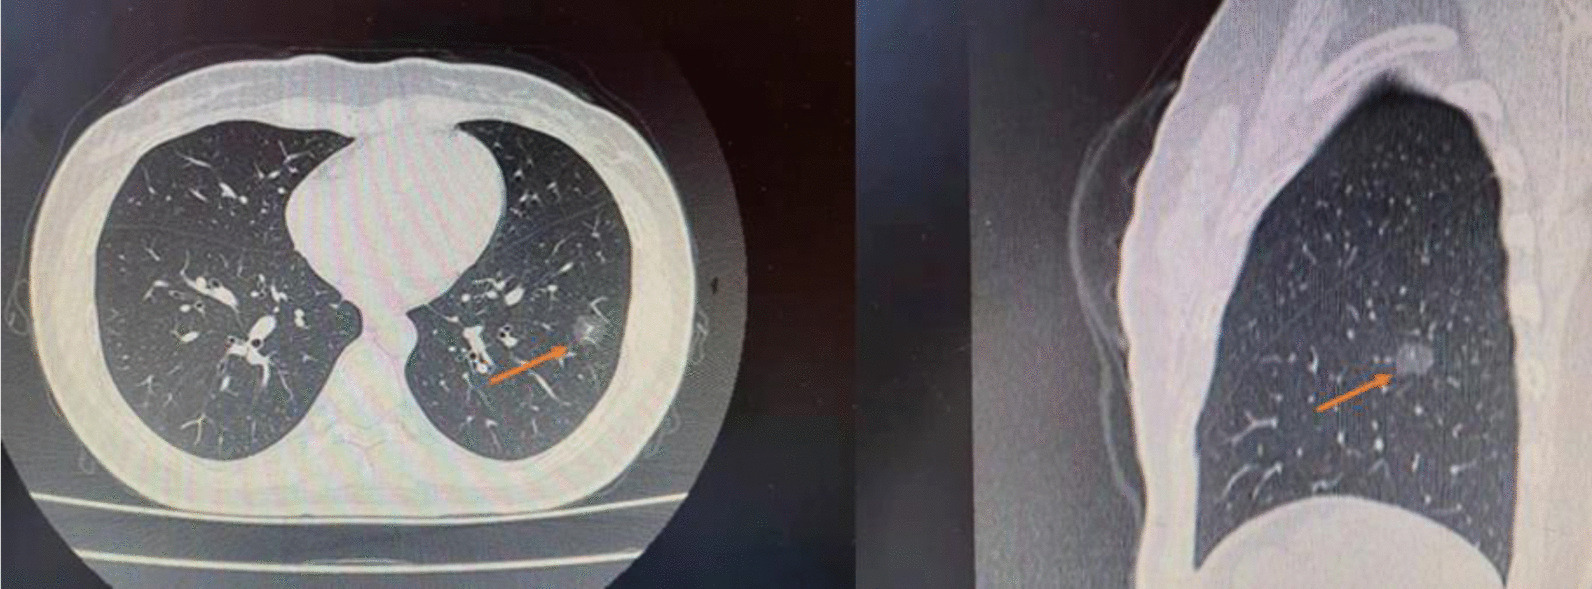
Computed tomography showed a 12 mm mixed ground glass opacity nodule (arrow) that was centrally located in the number 8 segment of the left lung (Left, axial plane; Right, sagittal plane)
